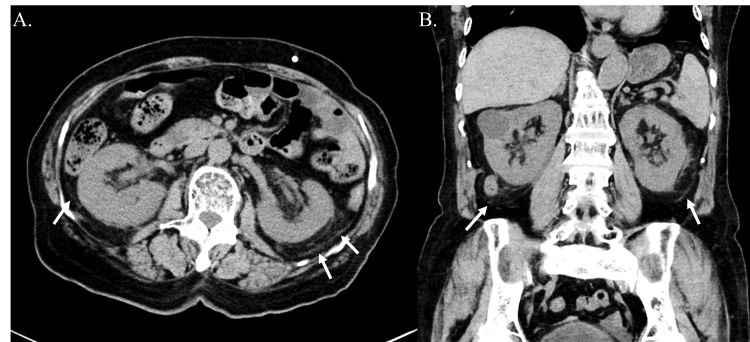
The renal computed tomography upon admission (A) Axial CT scan showing bilateral renal pelvic dilation, thickened renal pelvic walls, and inflammatory changes in perirenal fat appearing as cord-like opacities (arrows). The contrast was adjusted for better visualization. (B) Coronal CT scan confirming the bilateral nature of these findings, with evident renal pelvic wall thickening and surrounding inflammatory stranding in the perirenal fat tissue (arrows). The contrast was adjusted for better visualization.
